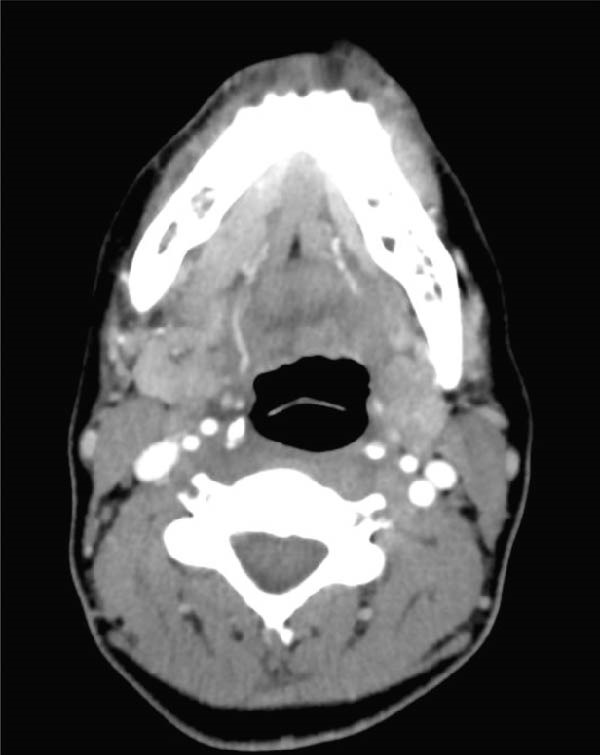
Contrast‐enhanced CT of Case 2 demonstrating a collection in the right pterygomandibular space.
